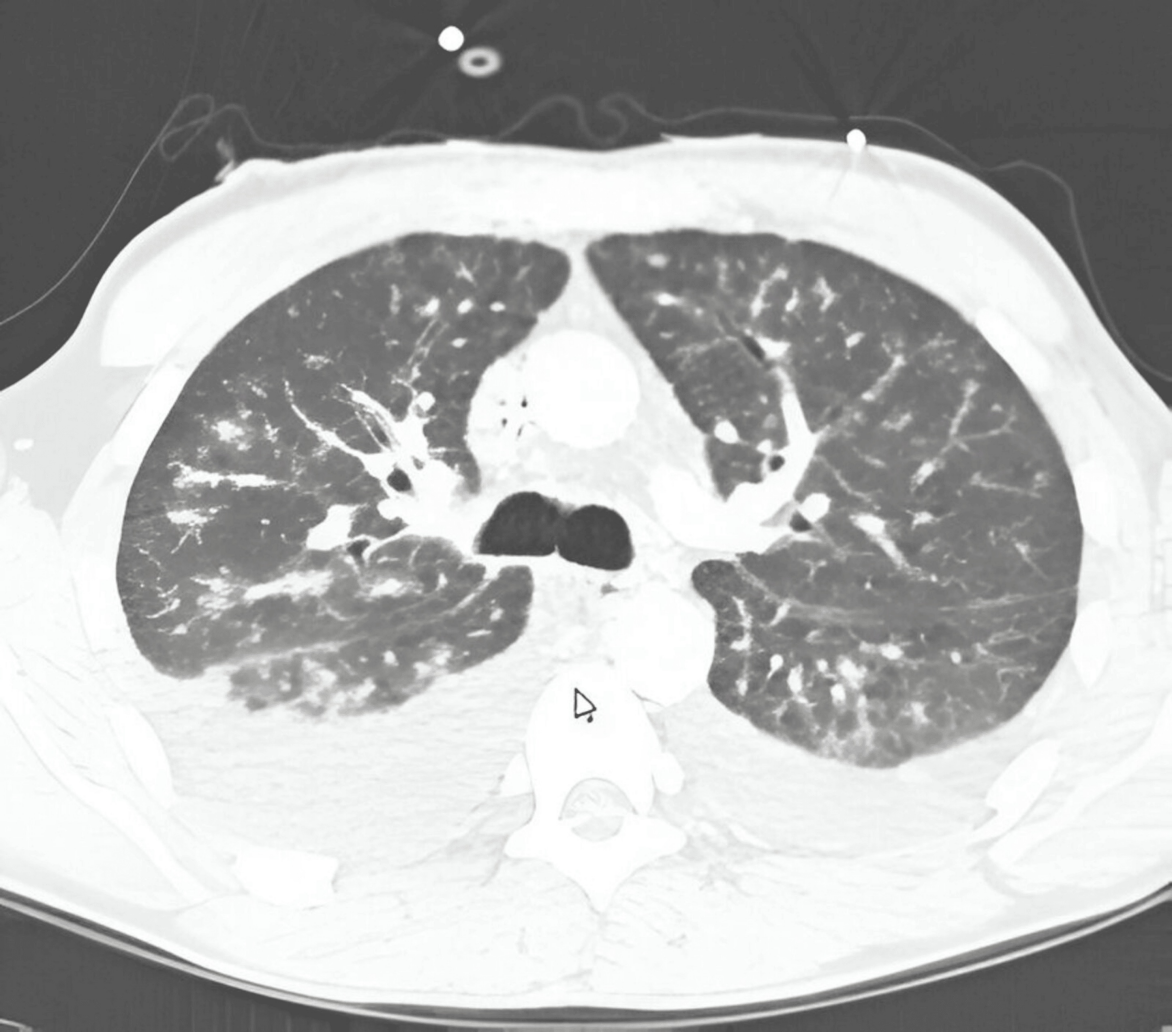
CT scan of the chest showing bilateral pleural effusions with patch consolidation.
